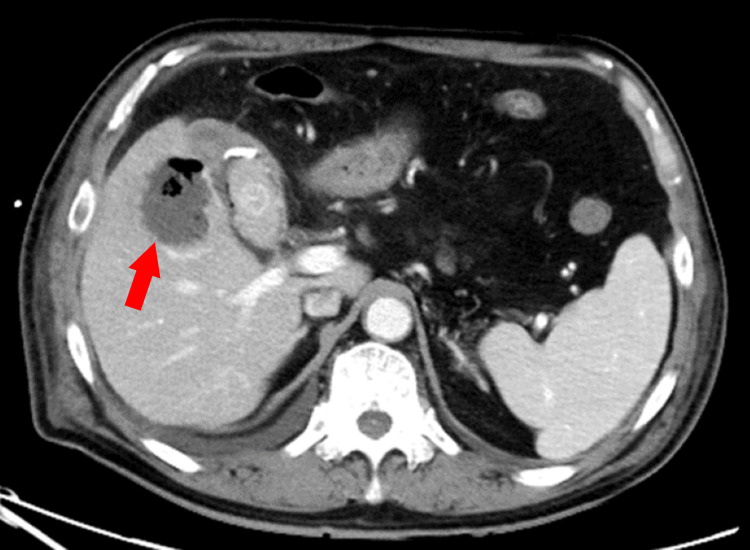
Abdominal CT scan showing fluid retention where there was originally a gas image of the liver abscess (red arrow).
